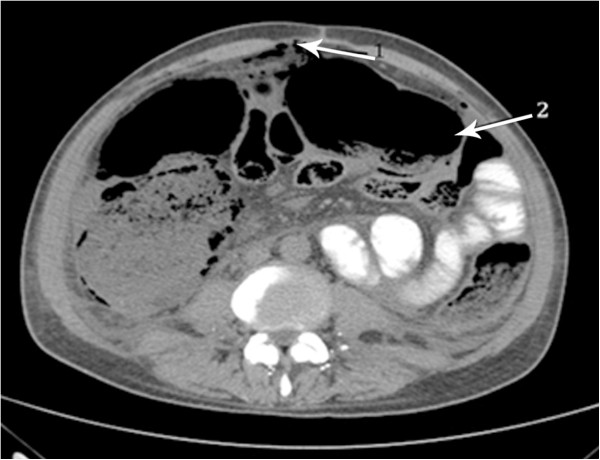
Pneumoperitoneum (arrow 1), gaseous distension of the colon (arrow 2) and the presence of free fluid in the abdominal cavity is shown in the abdominal CT.
